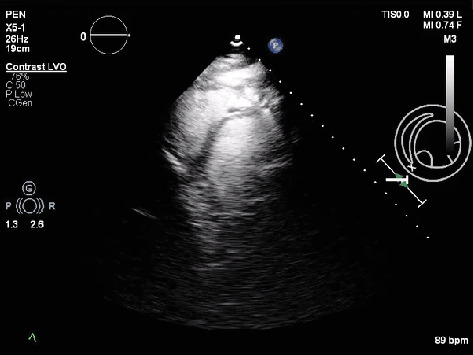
Echocardiogram showing septal bounce (short axis view).
